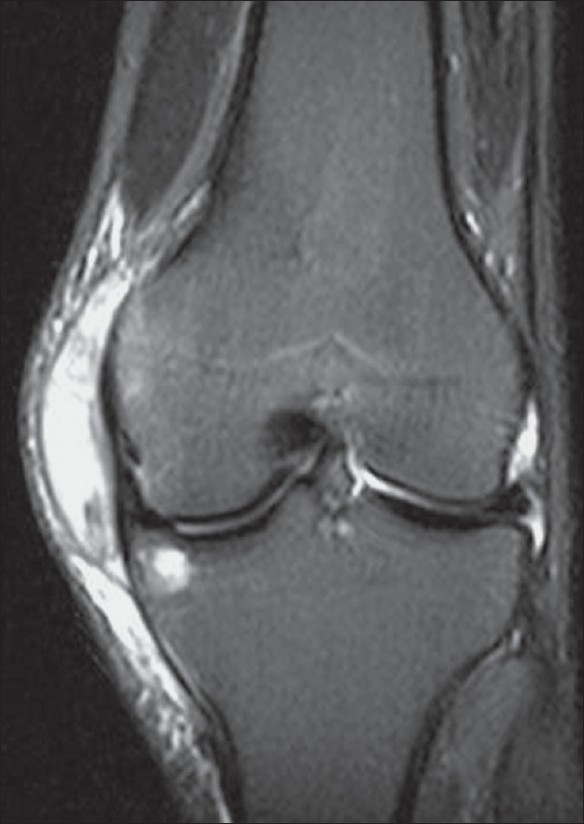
Soft tissue tuberculosis along the medial aspect of the left knee. There is associated osteomyelitis. Coronal, fat-suppressed, T2W MRI shows a high-signal-intensity soft tissue abscess, with adjacent bone marrow edema involving the medial condyle and a T2 hyperintense focus in the medial proximal tibia, due to the associated osteomyelitis
